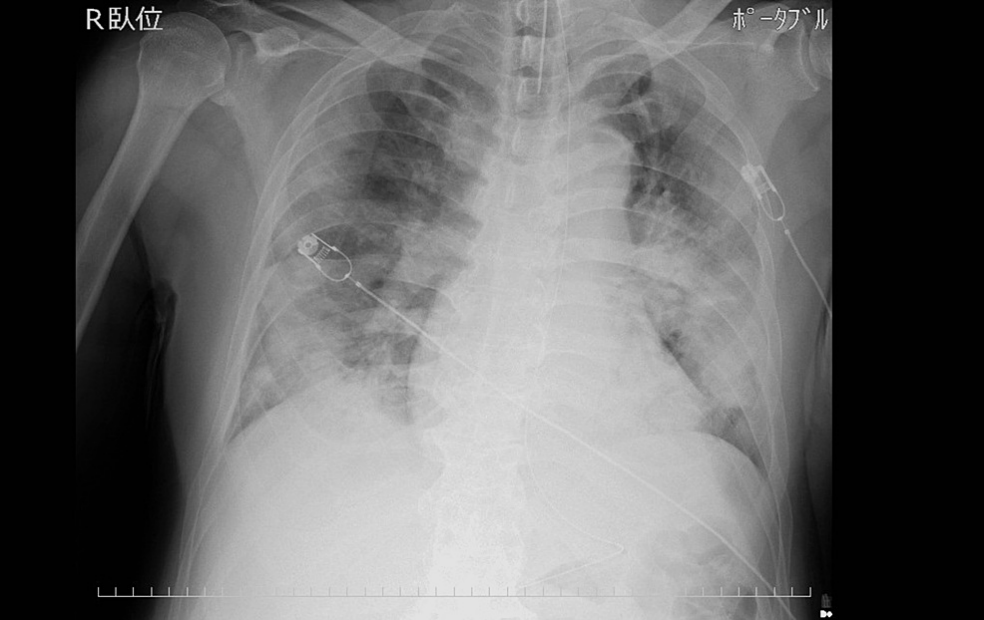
Chest x-ray at the time of admission. The chest x-ray reveals consolidation that is distributed bilaterally, reflecting severe respiratory decompensation due to COVID-19.
